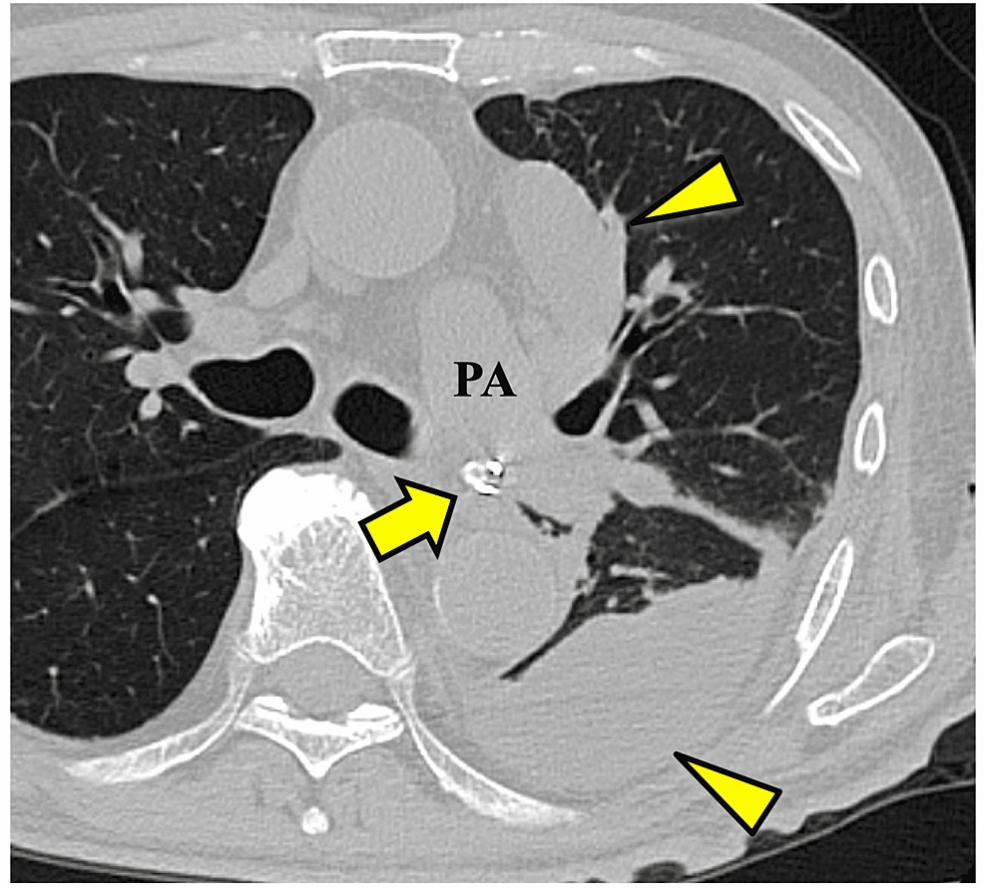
Preoperative chest computed tomography finding The chest tube protruding into the left lower lobe. The tip of the chest tube is placed near the left main bronchus and pulmonary artery (arrow). Multiple pyothoracic cavities are present (arrowhead). PA: pulmonary artery
